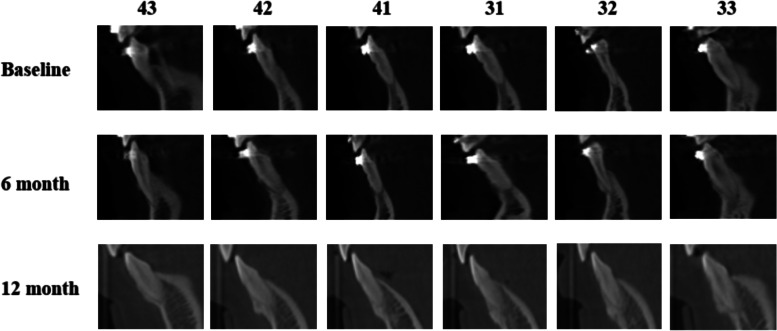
CBCT images of mandibular anterior teeth before surgery (baseline) and after surgery (6 months and 12 months)
